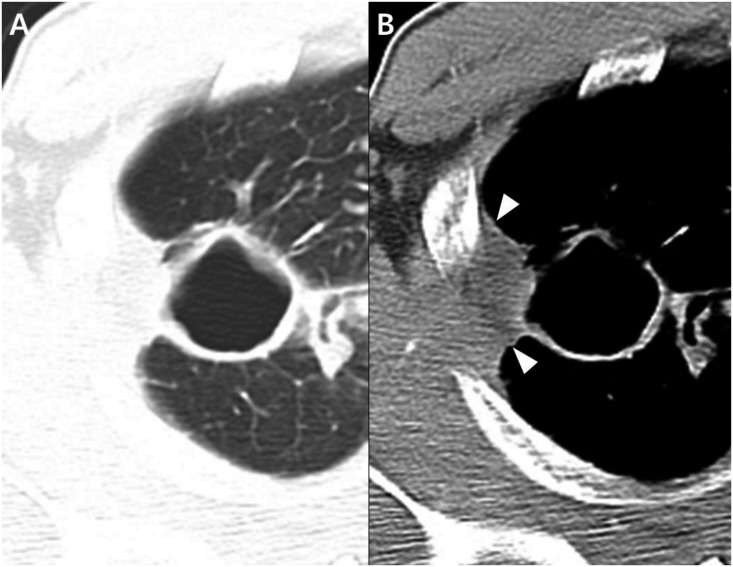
CT images of a *Mycobacterium intracellulare* pulmonary infection in a 41-year-old woman. Chest CT axial images with lung and mediastinal window settings (2.5-mm slice) show a thin-walled cavity (A) with adjacent pleural thickening (B) (arrowheads).
